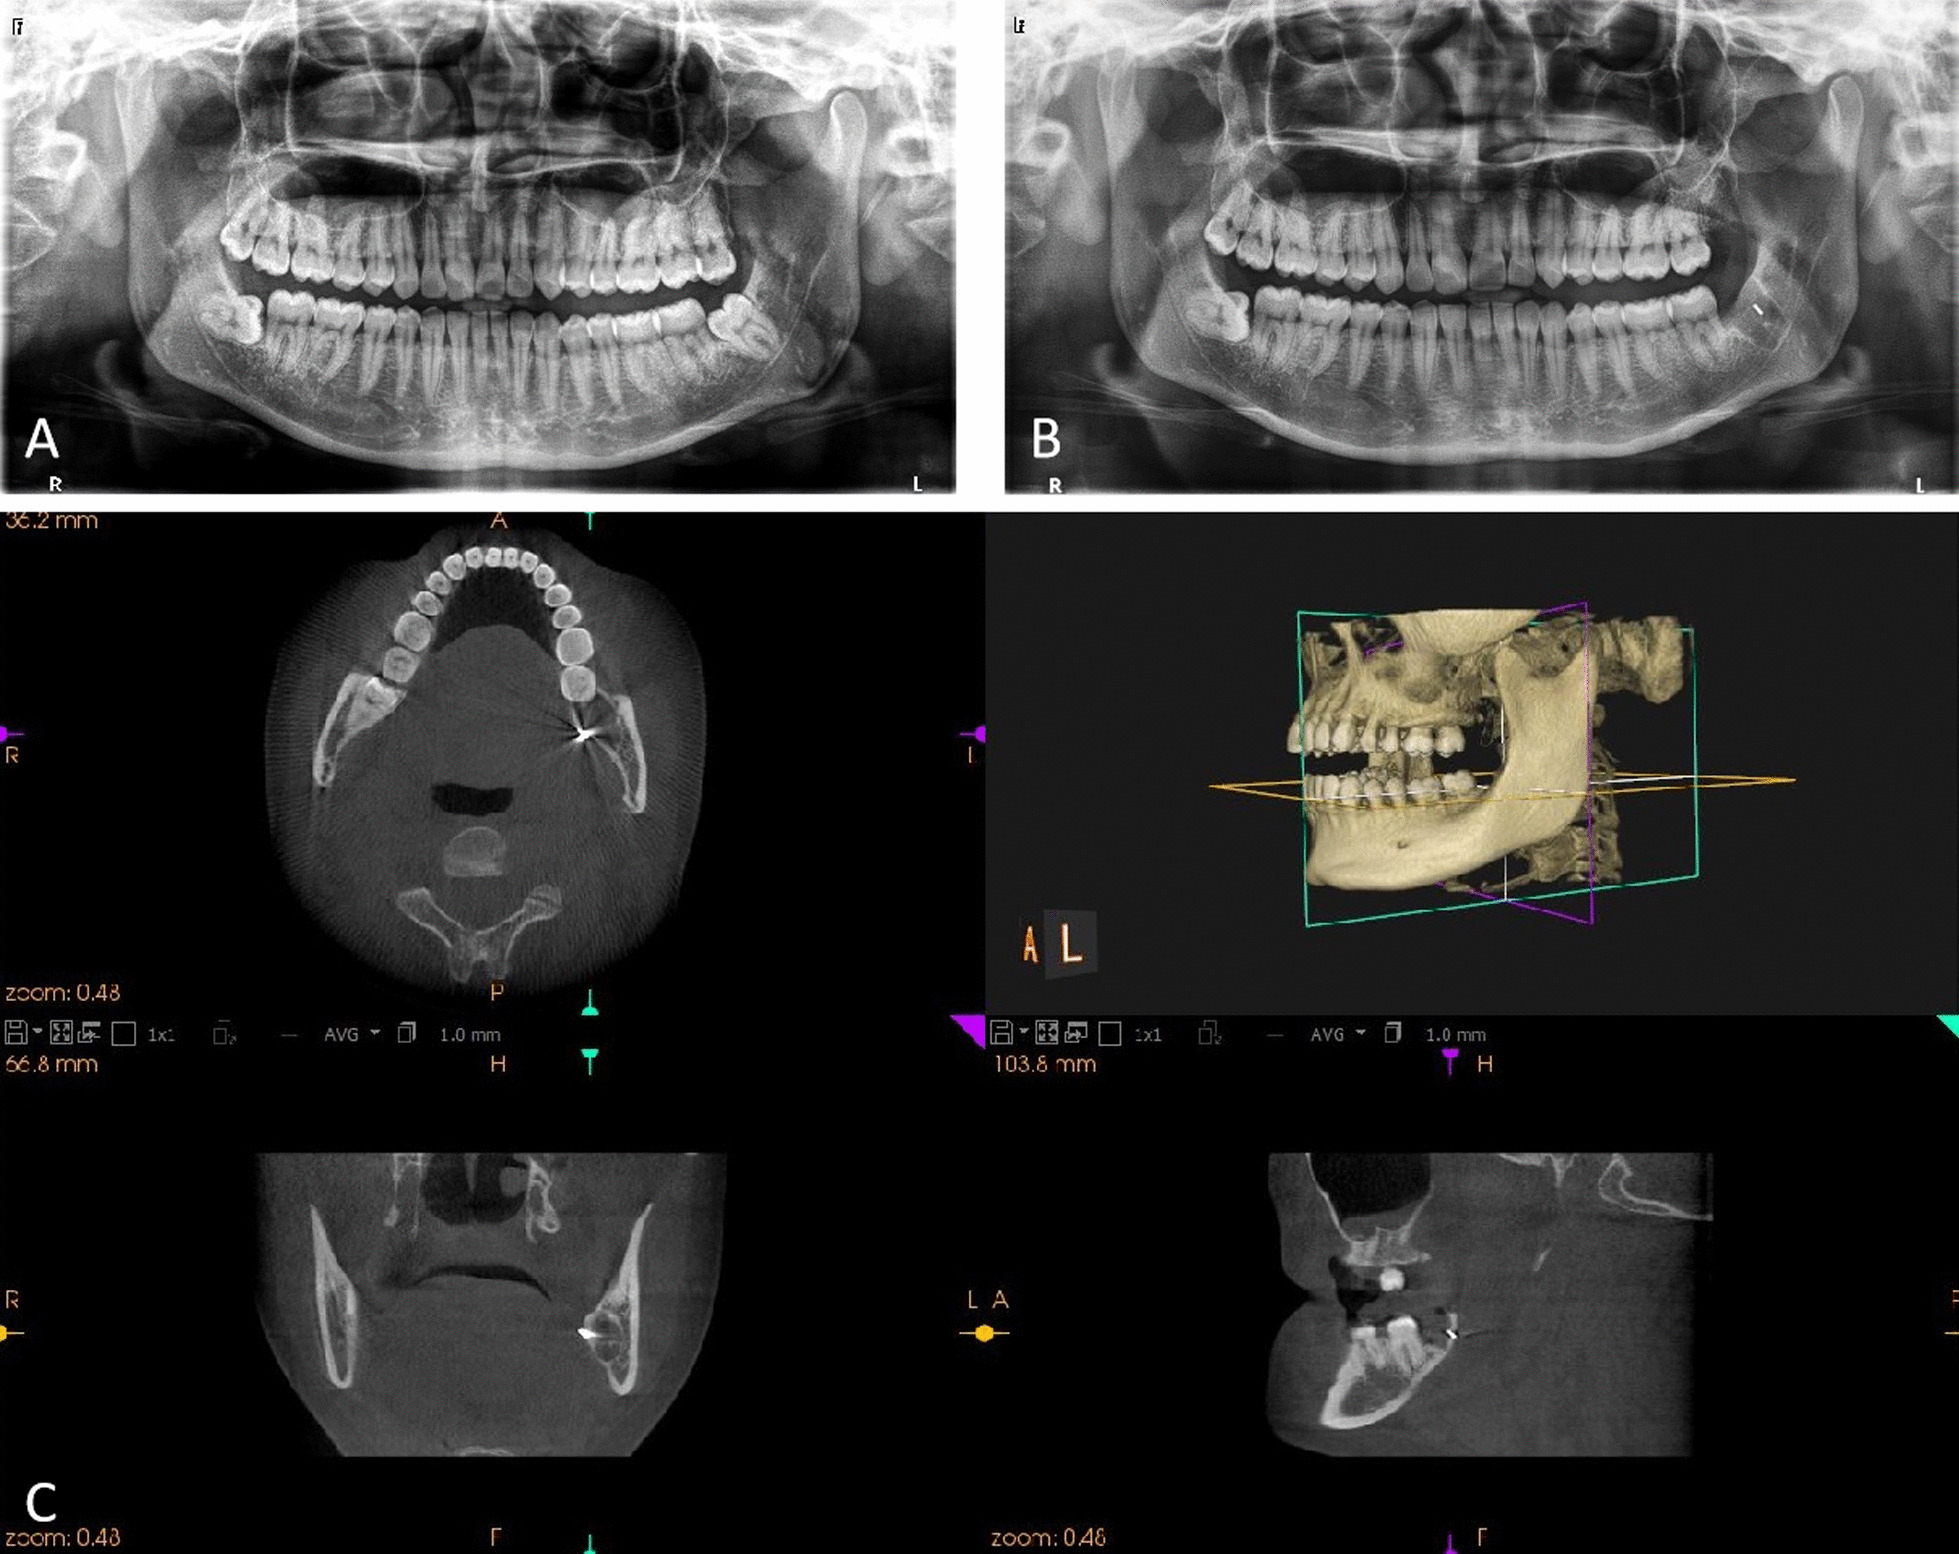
Case 2* A Preoperative panoramic radiograph. B Panoramic radiograph showing the bur fragment in the socket of the left mandibular third molar. C CBCT showing the 3D position of the bur fragment
